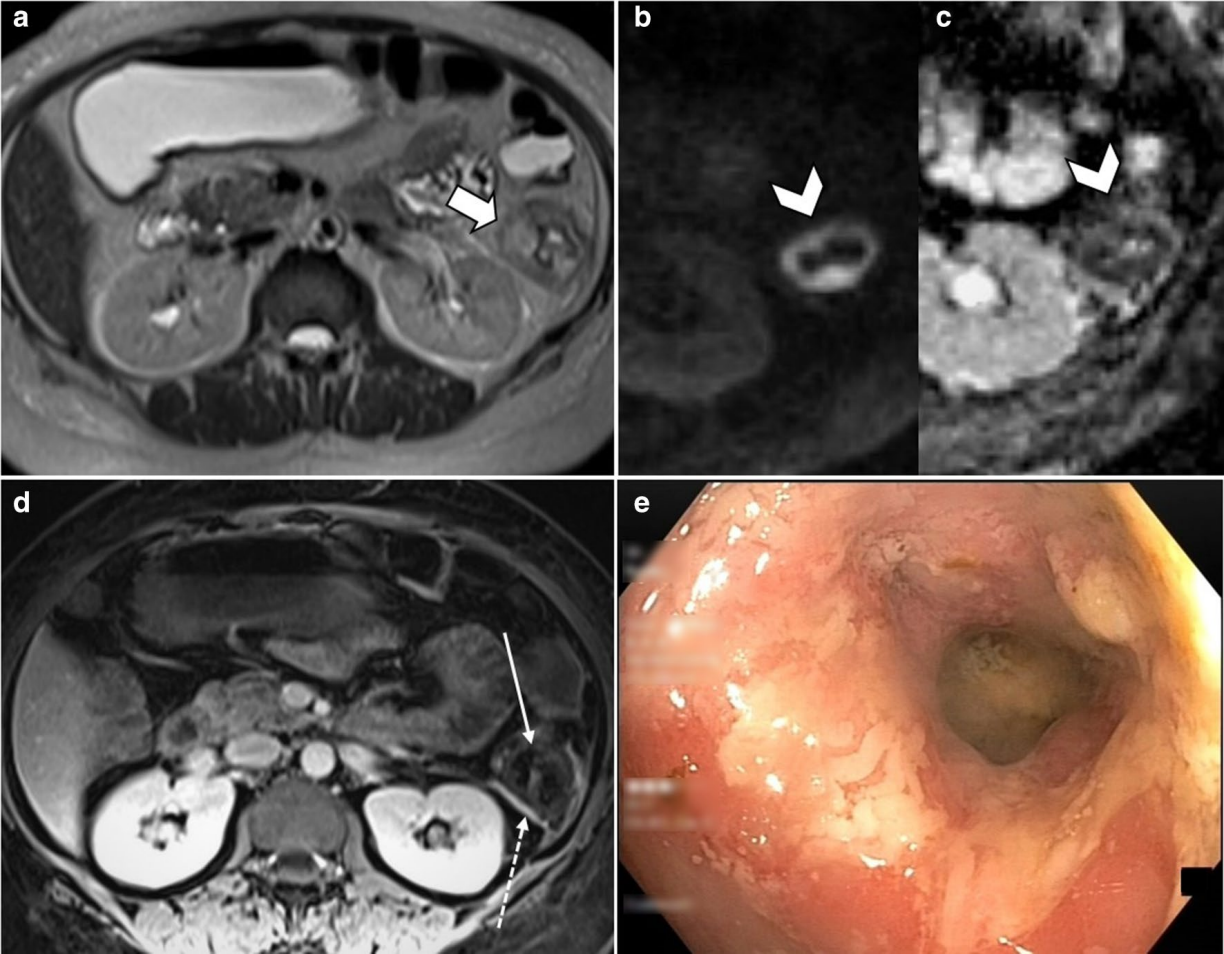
A 37 y/o female with known Systemic Lupus Erythematosus (SLE), presented with abdominal pain and diarrhea. a Axial T2-W sequence shows focal circumferential mural thickening (thick arrow) of the descending colon. Intense bowel wall diffusion restriction is noted on DWI (b) and ADC (c) sequences (arrowheads). On the post contrast axial T1-W image (d), there is lack of mural enhancement (thin arrow), favoring Lupus-induced ischemic colitis (intermediate stage). Accompanying mild serosal enhancement (dotted arrow) suggests underlying inflammation secondary to vasculitis. e Colonoscopic view of the same segment demonstrated fragile and edematous mucosa with loss of vascularity
